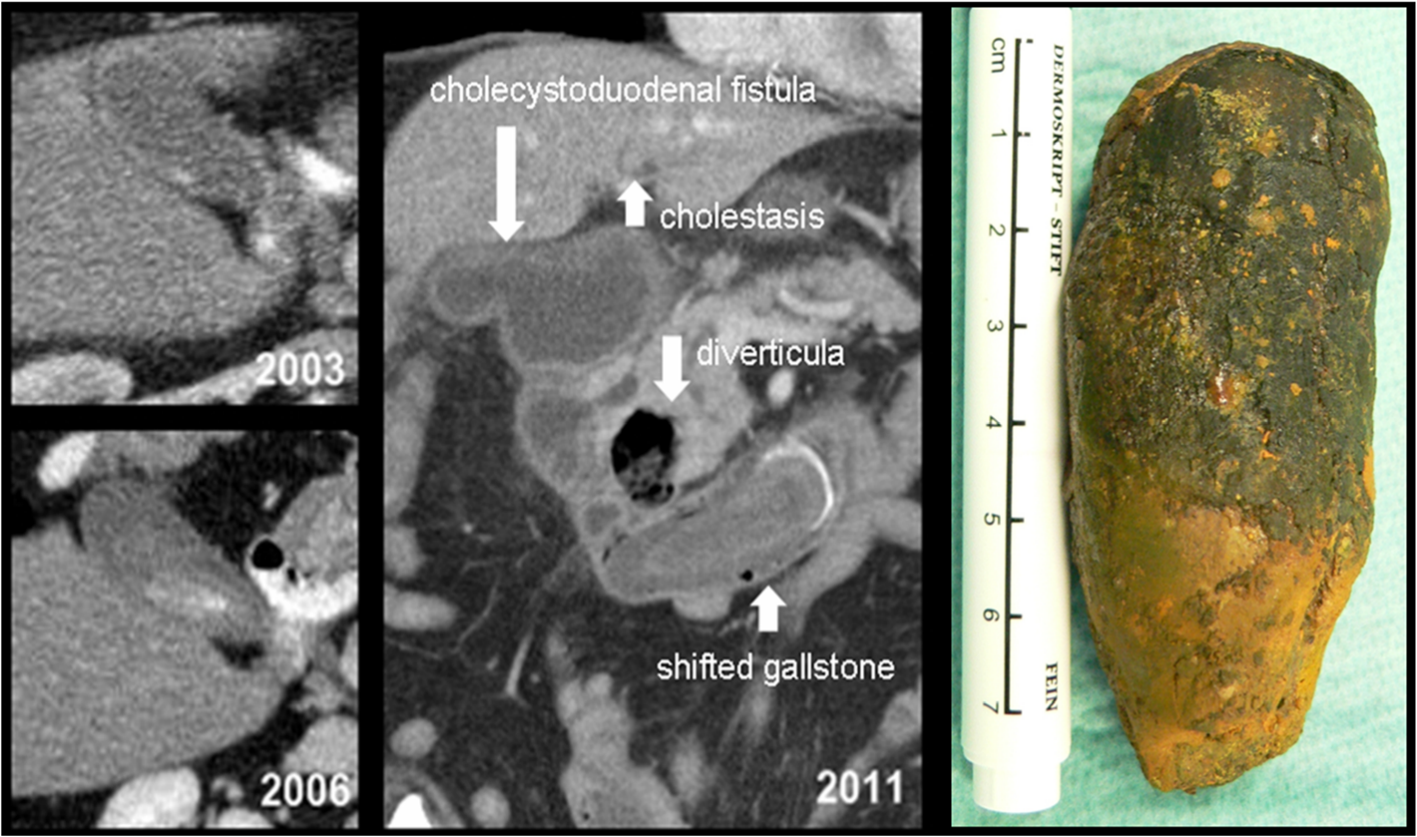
Case 1, axial slices of CT scans performed in 2003 and 2006 showing calculus development over time. Double-oblique MPR of the CT scan performed in 2011 at hospitalization, with delineation of the cholecystoduodenal fistula, the duodenal diverticula and the shifted gallstone. Retrieved gall stone on the right.
